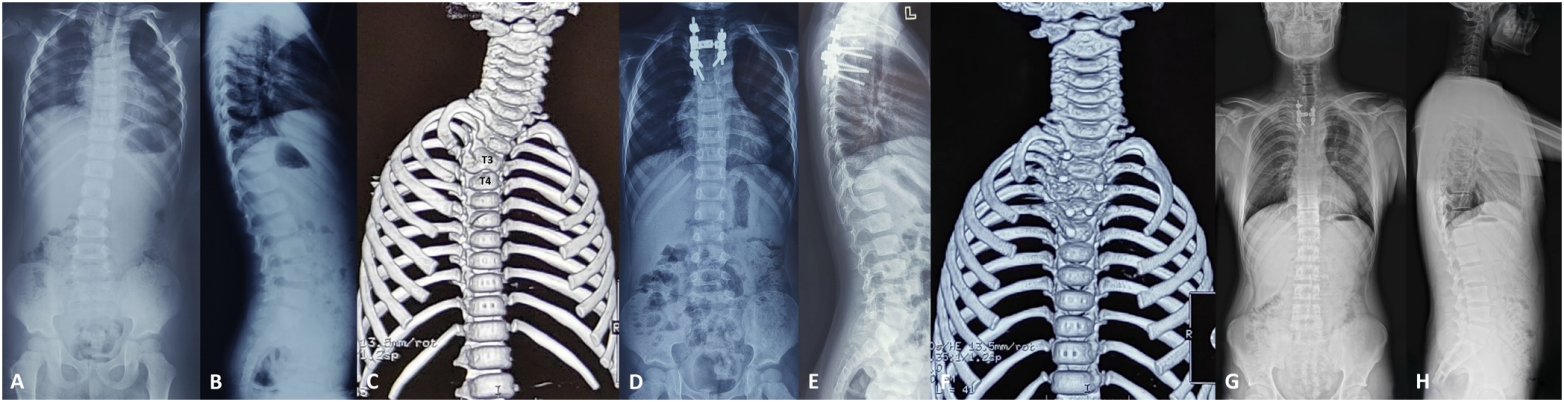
A 3-year-old boy (case 12) with congenital cervicothoracic scoliosis. (A,B) Preoperative radiographs showed that the segmental scoliosis was 41°, the distal compensatory curve was 8°, the T1 tilt was 23°, the clavicle angle was 8°, and the neck tilt was 5°. (C) Preoperative CT three-dimensional reconstruction showed the right T3-T4 hemivertebra. (D,E) Posterior T3-T4 hemivertebra resection combined with pedicle screw internal fixation was performed. Postoperative radiographs showed that the segmental scoliosis was 3° with a postoperative correction rate of 92.7%, the distal compensatory curve was 4°, the T1 tilt was 1°, the clavicle angle was 3°, and the neck tilt was 2°. (F) Postoperative CT demonstrated a solid fusion. (G,H) At the 13-year follow-up (the patient was 16 years old), the correction was maintained well, and no significant correction loss was observed during the follow-up.
